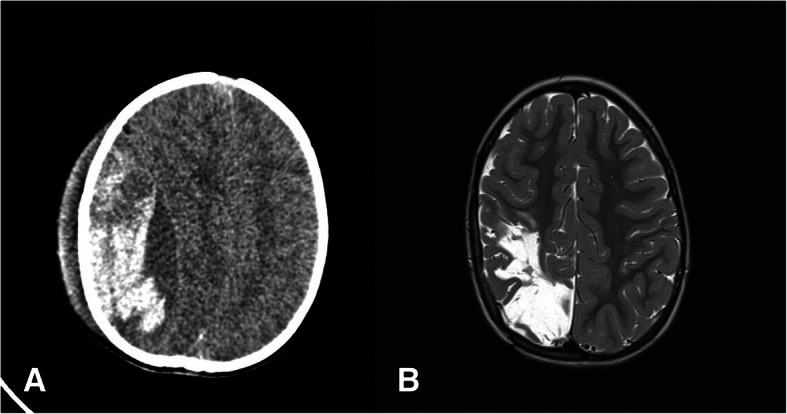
CT scan of the child with a large EDH (a) and the MRI performed 2 years after the trauma (b) showing an ischemic area in the territory of the right middle cerebral artery. Six months after surgery, the child still presented a severe hemiparesis, which resolved almost completely 3 years after the trauma
